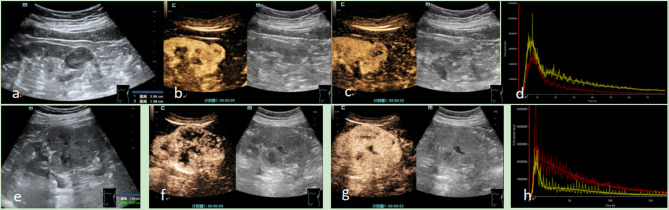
Representative CEUS images of nccRCC versus ccRCC. (a-d): A patient, female, 63 years old, with left chRCC. (a): Conventional ultrasound image displaying a hypoechoic mass in the left lower kidney, measuring 2.86 cm x 1.98 cm, with clear boundaries and a regular shape; (b): Contrast-enhanced ultrasound (CEUS) image during the perfusion phase at 9 s, demonstrating homogeneous low enhancement within the lesion and peripheral annular enhancement; (c): CEUS image during the washout phase at 52 s, depicting low enhancement within the lesion and peripheral ring enhancement; (d): Time-intensity curve (TIC) of nccRCC showing slow advance, fast retreat and low enhancement. (e-h): A patient, female, 72 years old, with left ccRCC. (e): Conventional ultrasound image displaying a hypoechoic mass in the left middle kidney, measuring 7.55 cm x 5.43 cm, with clear boundaries and a regular shape; (f): Contrast-enhanced ultrasound (CEUS) image during the perfusion phase at 9 s, demonstrating Heterogeneous high enhancement within the lesion and peripheral annular enhancement; (g): CEUS image during the washout phase at 52 s, depicting high enhancement within the lesion and peripheral ring enhancement; (h): Time-intensity curve (TIC) of nccRCC showing fast advance, slow retreat and high enhancement
